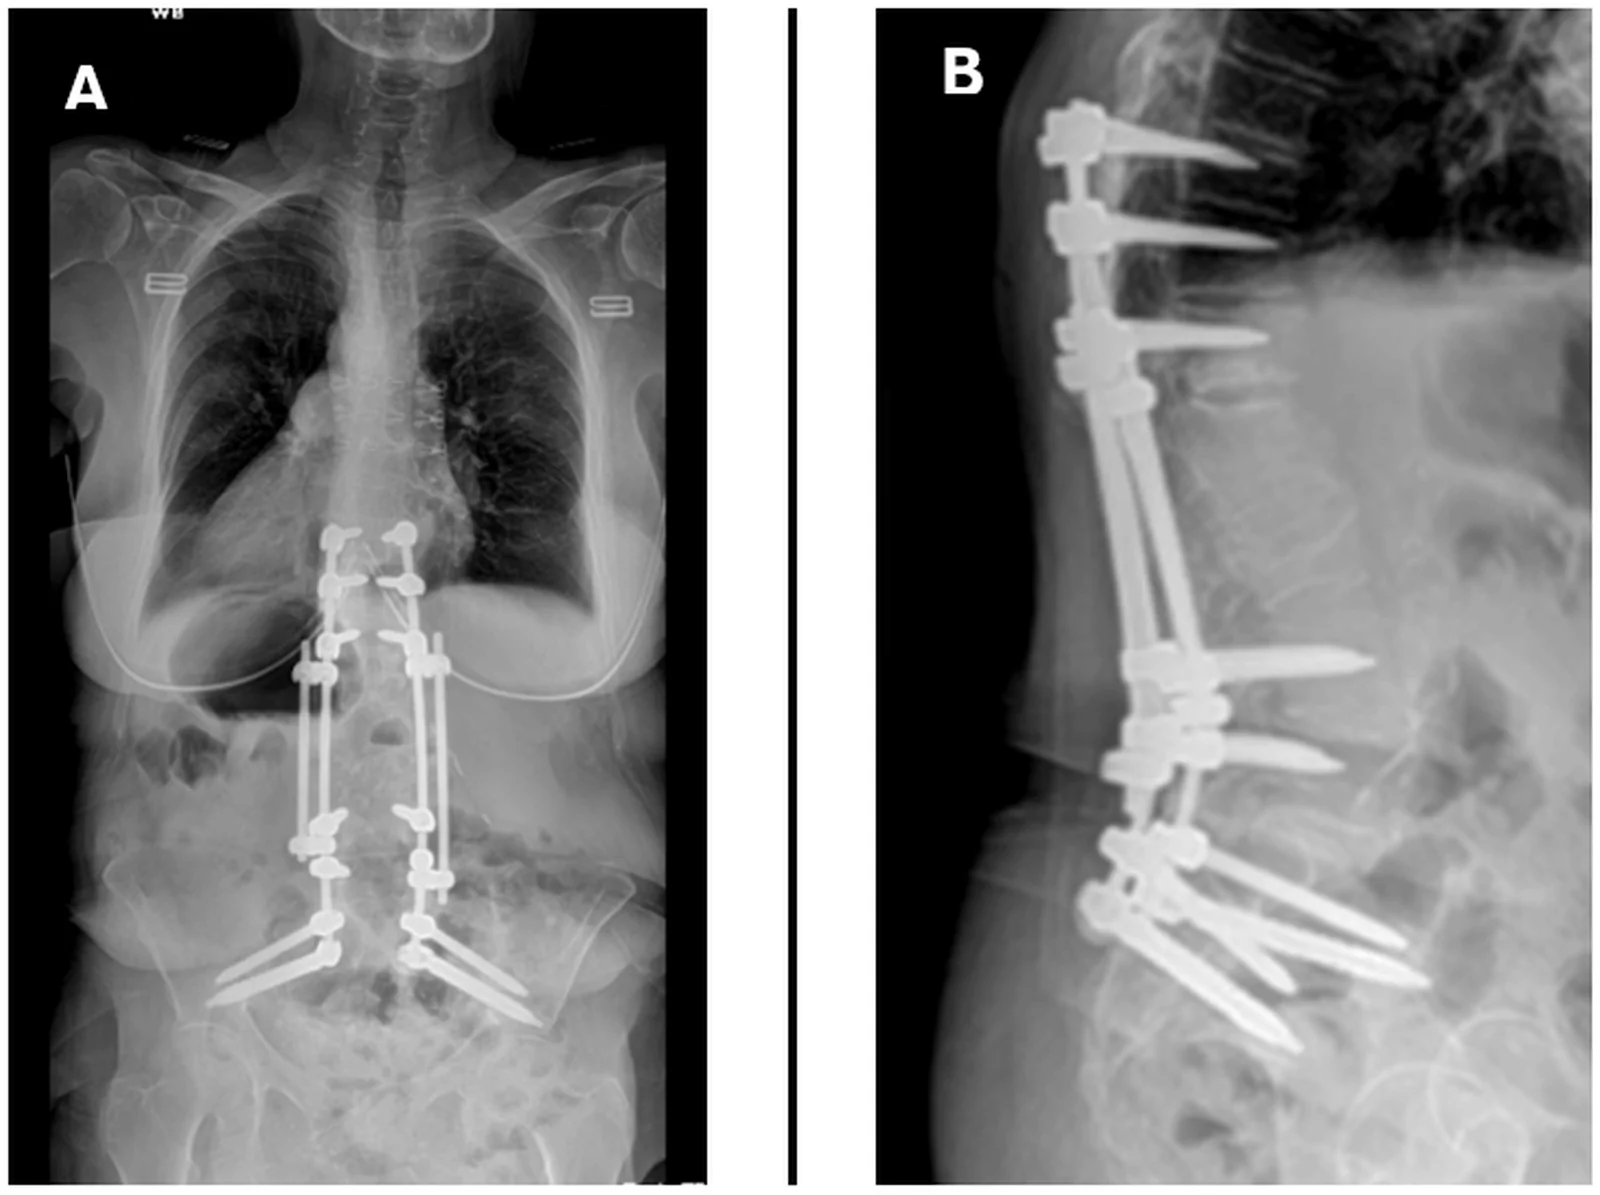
(A) Postoperative standing anteroposterior radiograph and (B) lateral radiograph demonstrating the long-segment T10–pelvis quad-rod construct and improved sagittal alignment.
